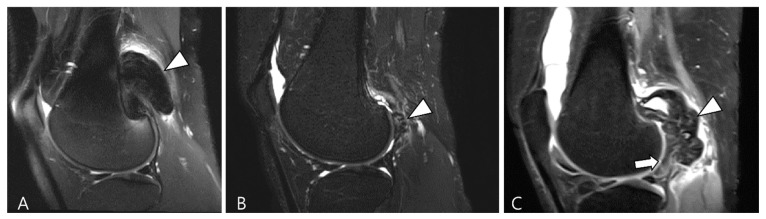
Serial T2WI follow-ups for D-TSGCT. (A) Initial MRI shows a low SI mass at the popliteal fossa (arrowhead). (B) After En bloc excision, the first follow-up MRI (1 year) shows small low SI nodule at the posterior joint capsule (arrowhead). (C) Second follow-up MRI (3 years) shows recurrent mass extra-articularly (arrowhead) and extensive nodular thickening intra-articularly (arrow).
